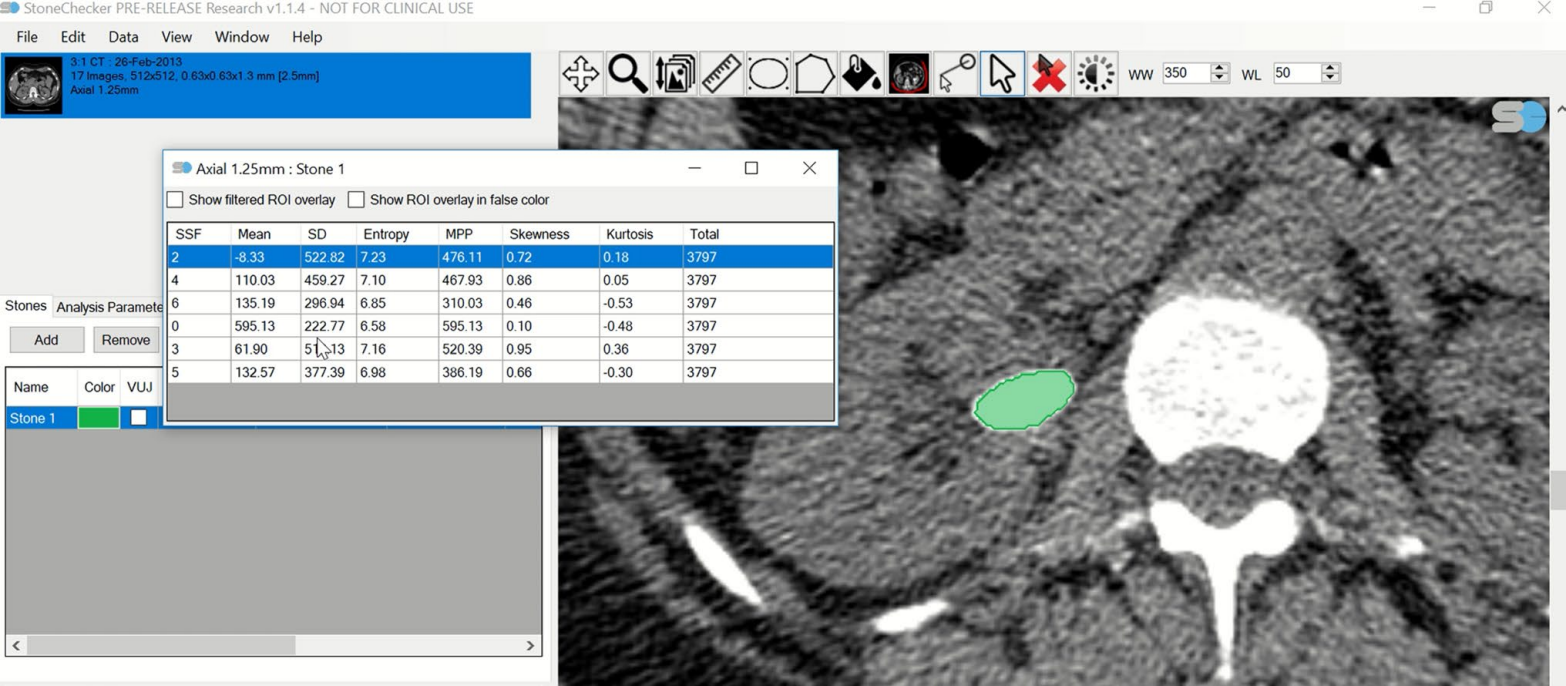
Example of the generation of computed tomography textural analysis variables using the StoneChecker Software. The green area represents the semi-automated region of interest generated by the software
